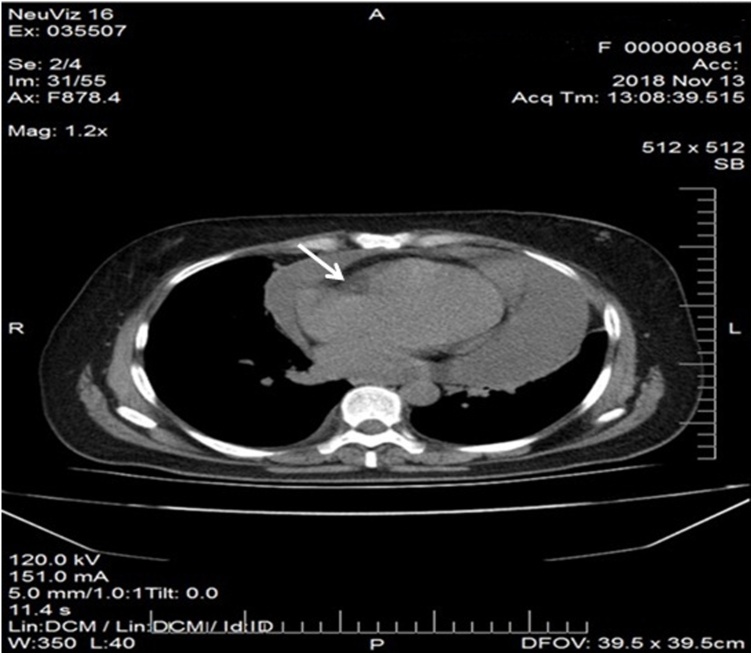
Computed tomography image of large pericardial effusion and pericardial mass in pericardial fat tissue (enlargement of pericardial lymph node?) (arrow).
